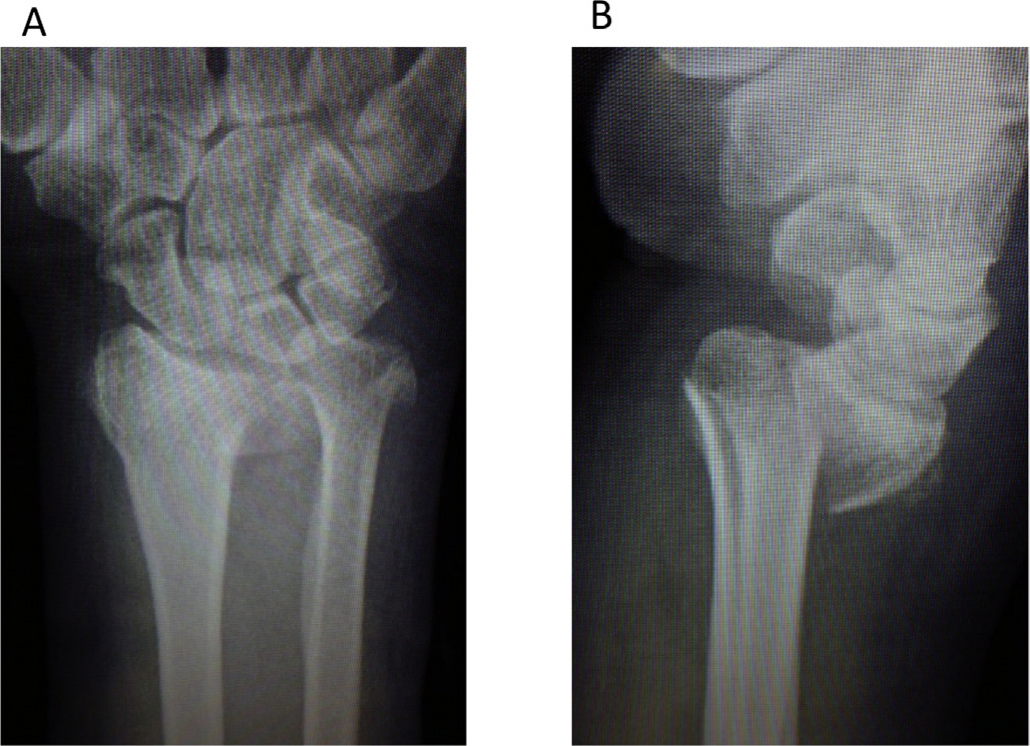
Plain radiography at the time of injury. A: The dorsal dislocated left distal radius fracture associated with ulnar styloid process fracture was observed with a frontal view. B: Volar dislocation of the ulnar head was observed with a lateral view.
